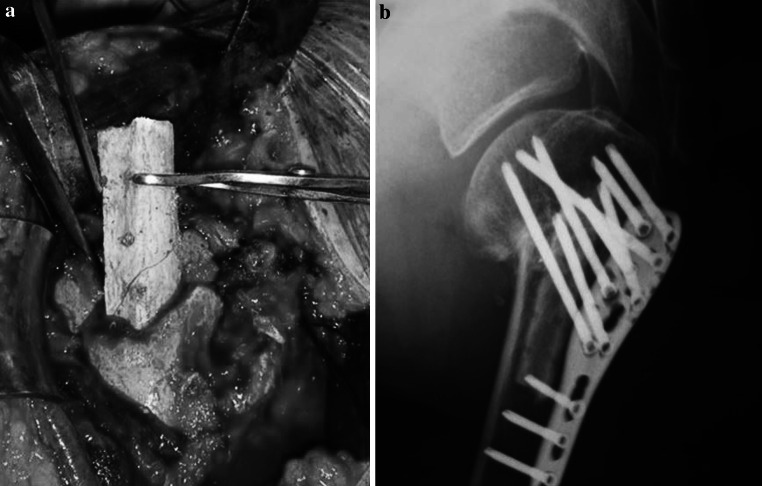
a Cortical strut autograft from fibula in a proximal humeral non union treated by ORIF. b One year X-ray control show bone healing and the persistence of the autograft
